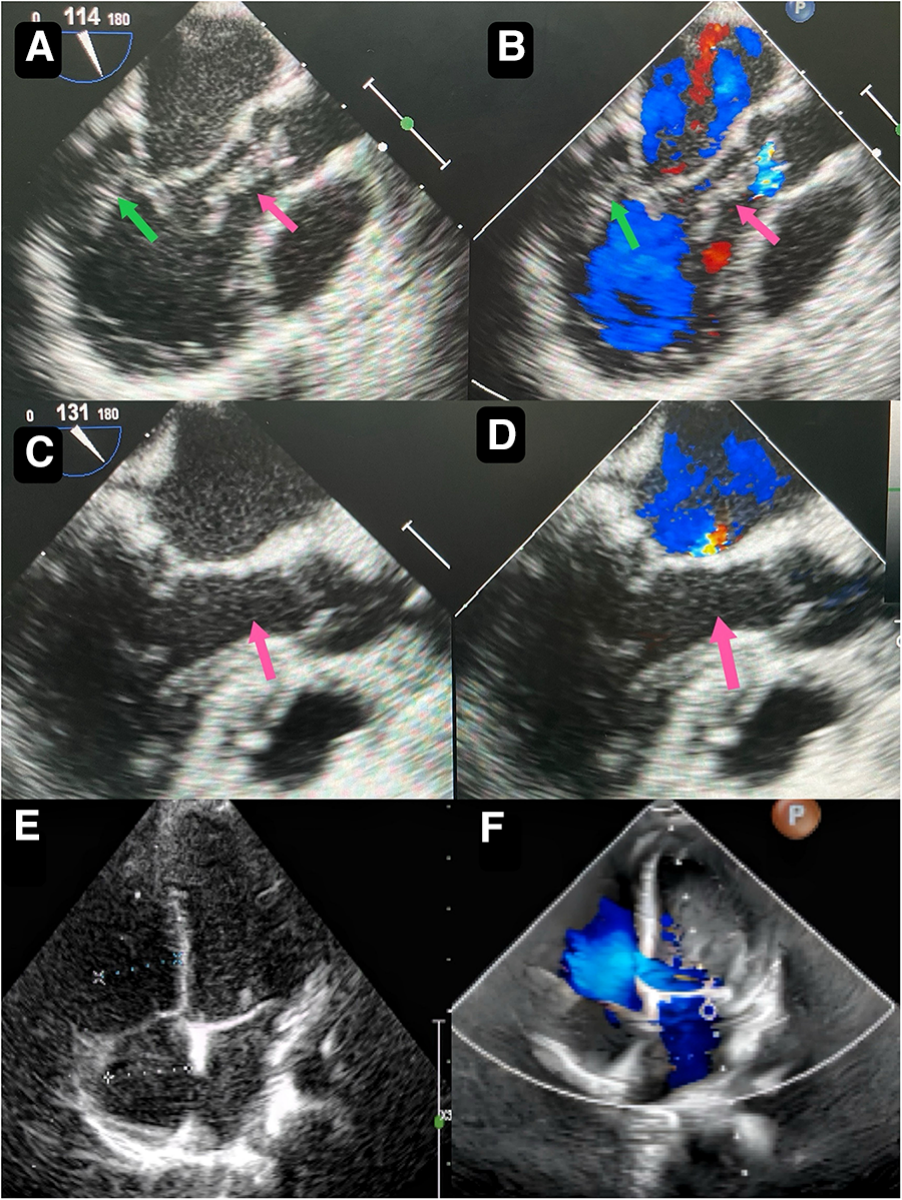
Preoperative transthoracic echocardiography reveals a slightly hyperechoic, approximately 32 mm by 16 mm mass in the posterior wall of the left ventricle (A) echogenic “string of beads” is observed wiggling in the left ventricular outflow tract, with one end connected to the posterior part of the left ventricle and the other end appearing to be connected to the left coronary sinus of the aorta (B) postoperative transesophageal echocardiogram shows complete removal of the mass (C) postoperative transesophageal echocardiogram shows competent mitral valve (D) preoperative transthoracic echocardiogram shows competent mitral valve (E) postoperative transthoracic echocardiogram mitral valve shows trivial regurgitation (F).
